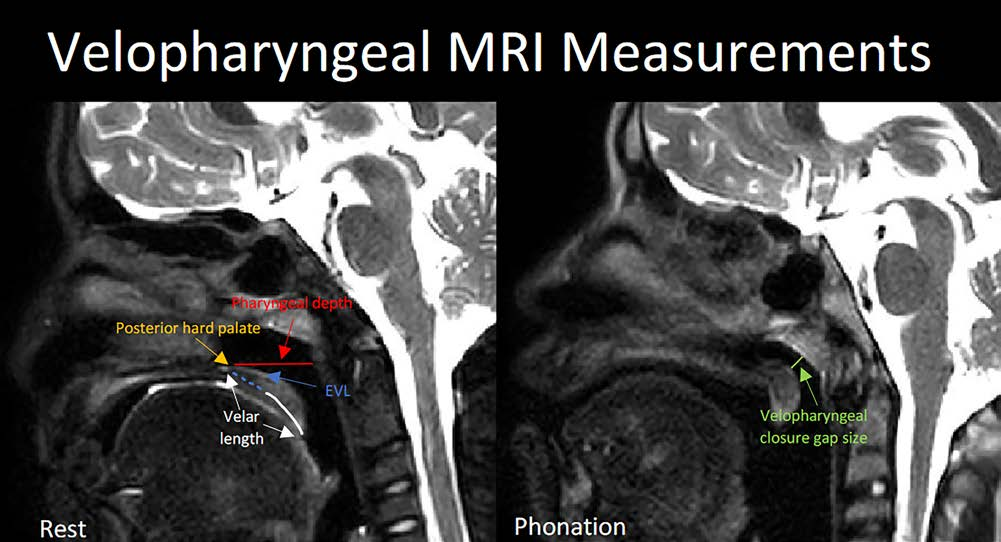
Velopharyngeal MRI measures at rest of pharyngeal depth in red, effective velar length (EVL) in blue, velar length in white, and velopharyngeal gap size during phonation in green. The posterior hard palate is identified in yellow.
